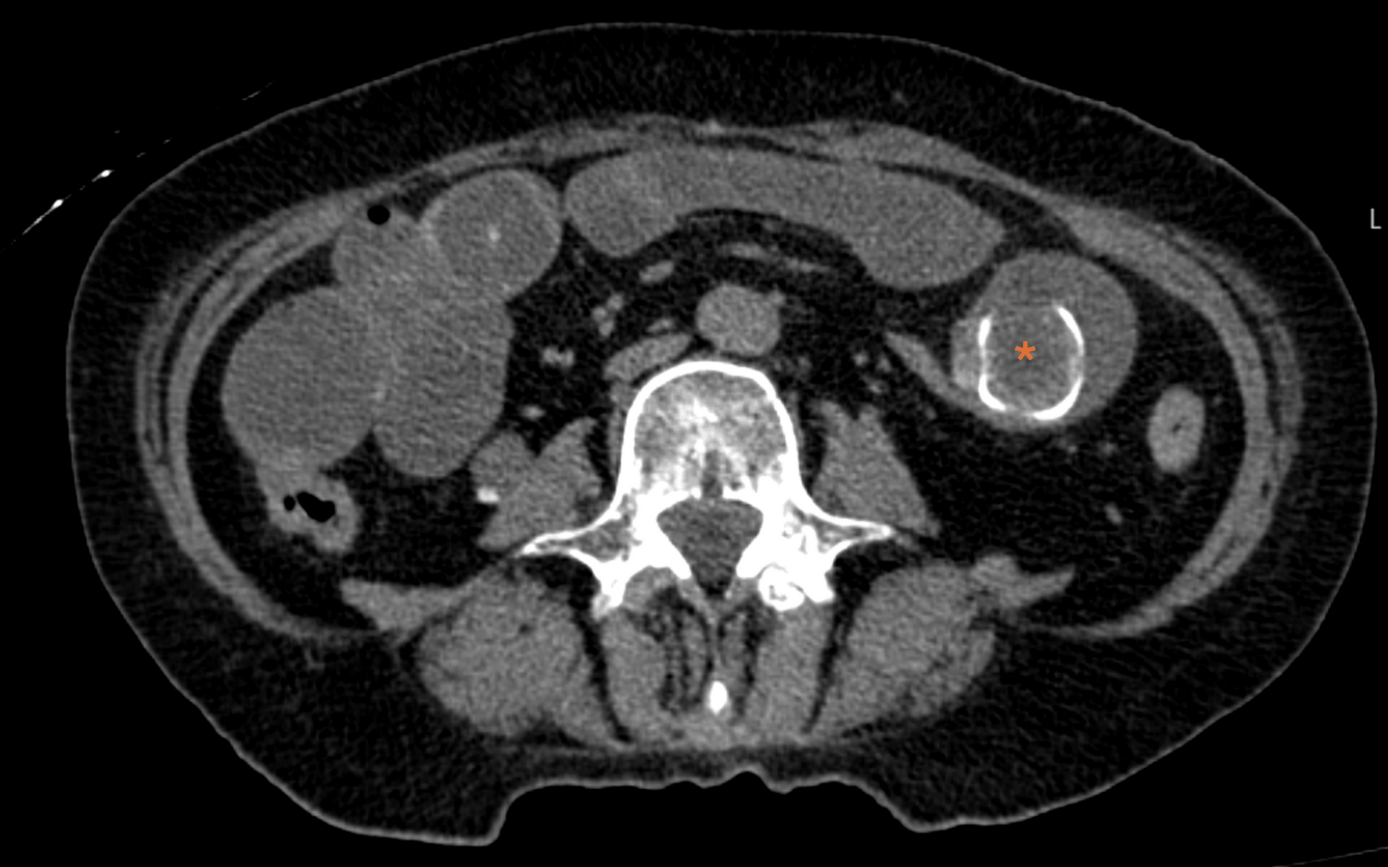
Non-contrast CT showing the migration of a mineralized biliary stone (asterisk) into the lumen of a jejunal loop. CT: computed tomography
